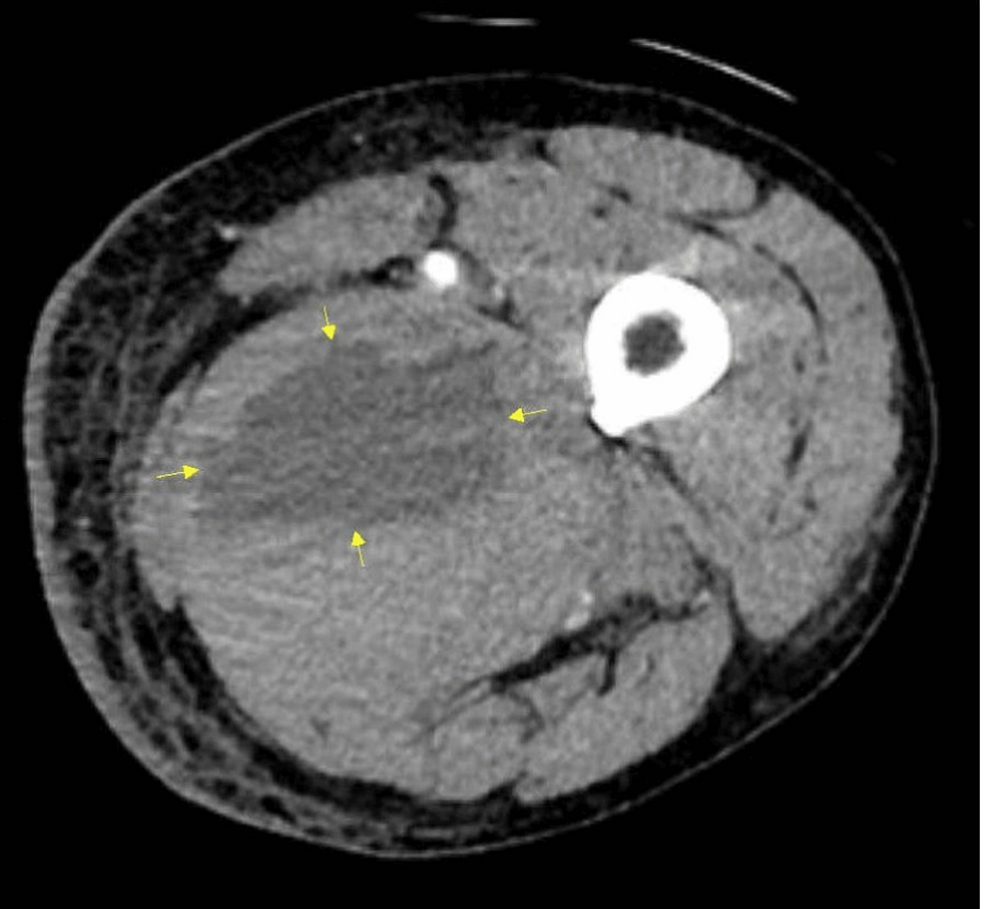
CT angiogram demonstrating hematoma within the adductor muscle.
